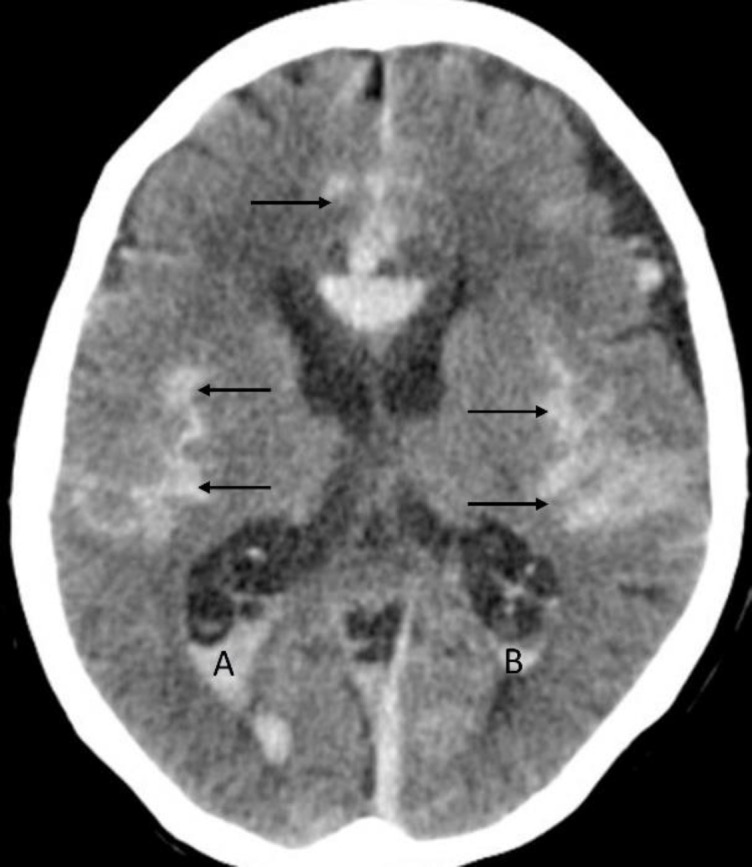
Non-contrast axial CT brain of a 67 years-old female with subarachnoid hemorrhage (arrows) and intraventricular extension in the occipital horns of the lateral ventricle bilaterally (A-B)
